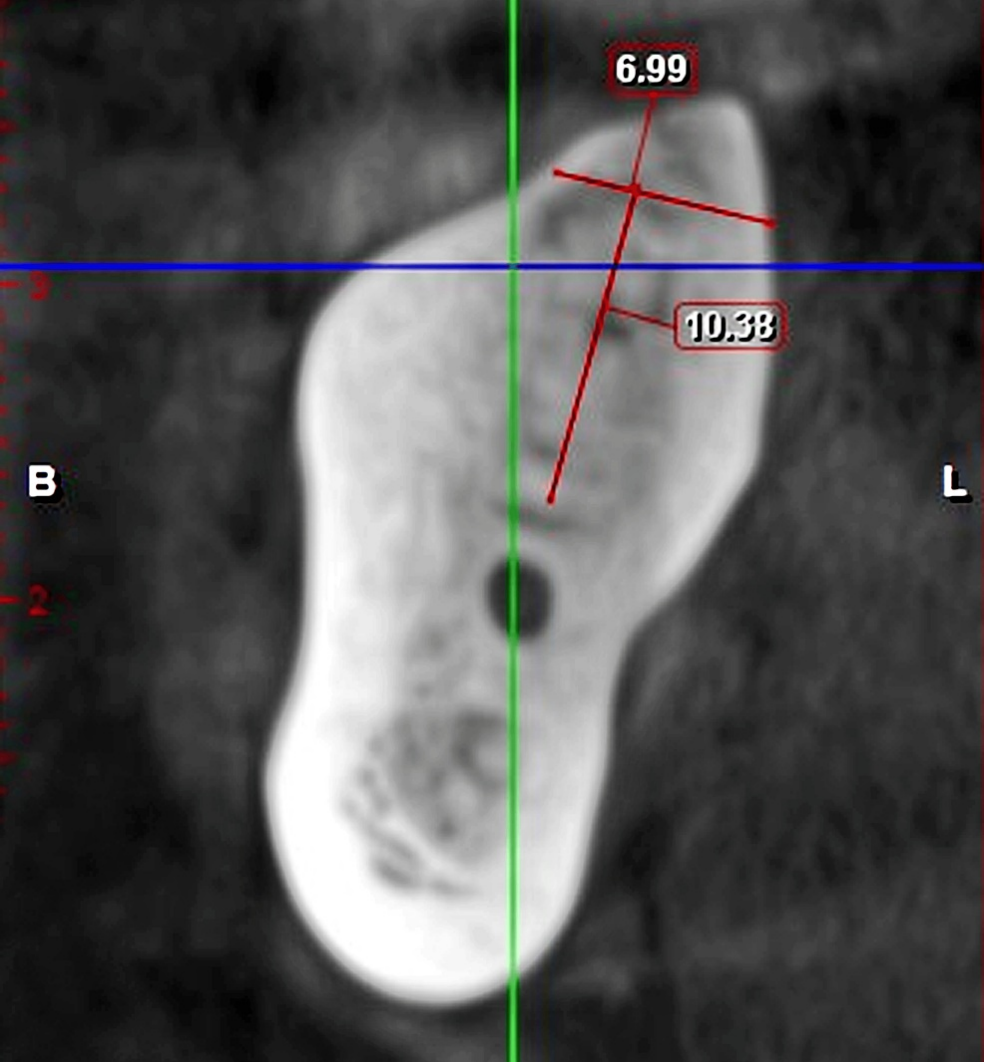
CBCT image 2 (sagittal section, 2 mm slice view) The cone beam computed tomography (CBCT) image shows available bone height and width in the 47 region.
